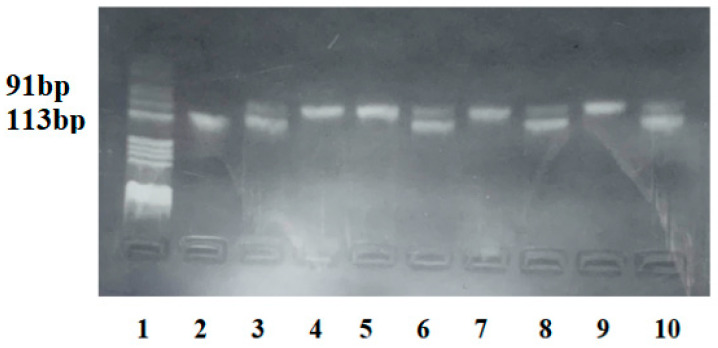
Agarose gel electrophoresis for identification of rs7903146 polymorphism. Lane 1—pBRHaeIII Digest DNA molecular marker; Lane 2—homozygous TT genotype: fragment of 113bp; Lanes 3, 6, 8, 10—heterozygous CT genotype: fragments of 113 and 91 bp; Lanes 4, 5, 7, 9—homozygous CC genotype: fragment of 91 pb.
